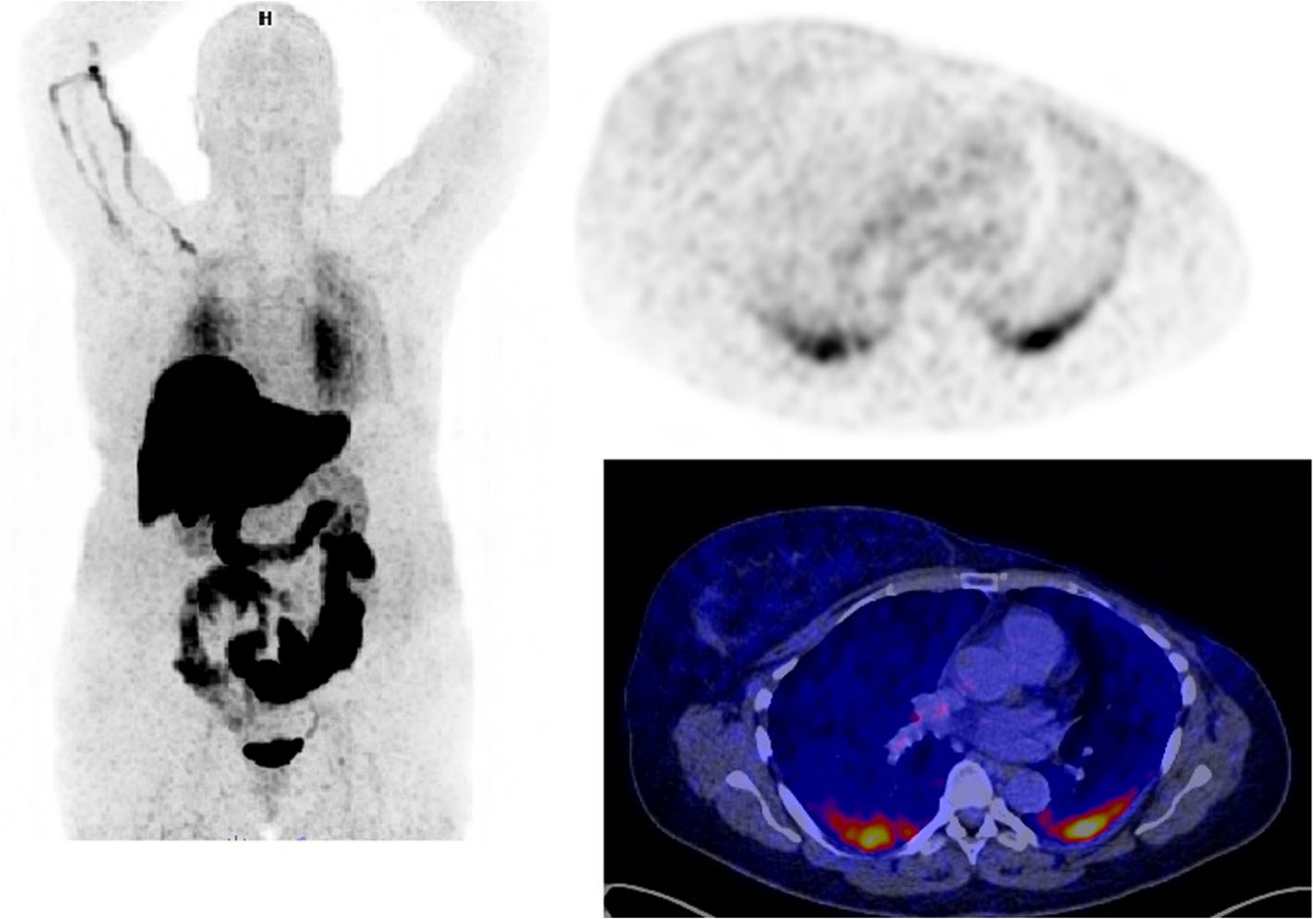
An example of a FES-PET/CT scan with high non-tumor uptake in the lungs
